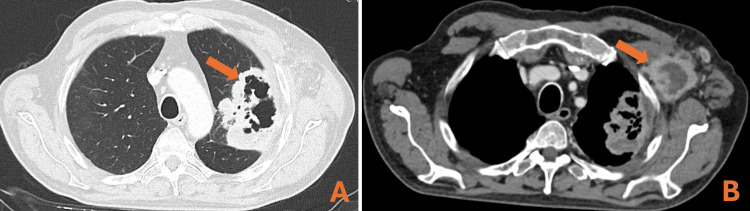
Response to treatment evaluation by CT scan demonstrating the increment of the lung lesion (A) and left axillary node (B) CT: computed tomography
